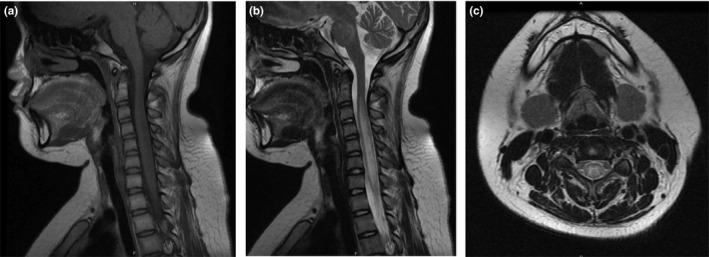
MRI‐scan 4 days after symptom onset. Cervical cord MRI image showing areas of T2 prolongation on the central spinal cord like H, and the cervical cord was swollen. (a) Sagittal T1. (b) Sagittal T2. (c) Axial T2
